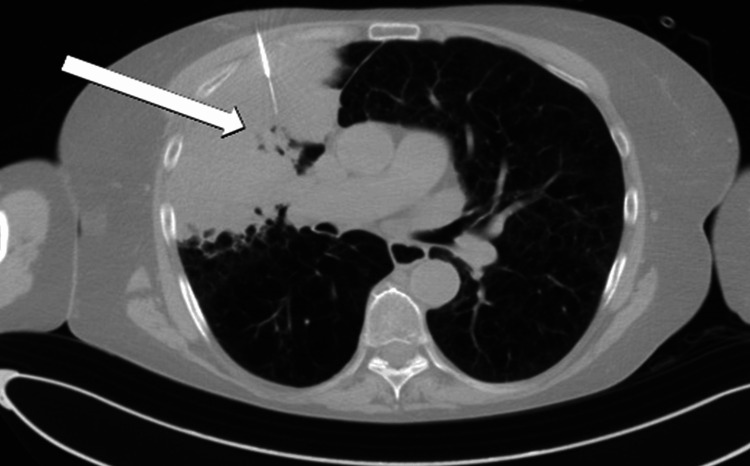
Computed tomography-guided percutaneous biopsy of the right upper lobe consolidation The white arrow indicates the part of the tumor within atelectasis that was biopsied confirming pulmonary adenocarcinoma.
